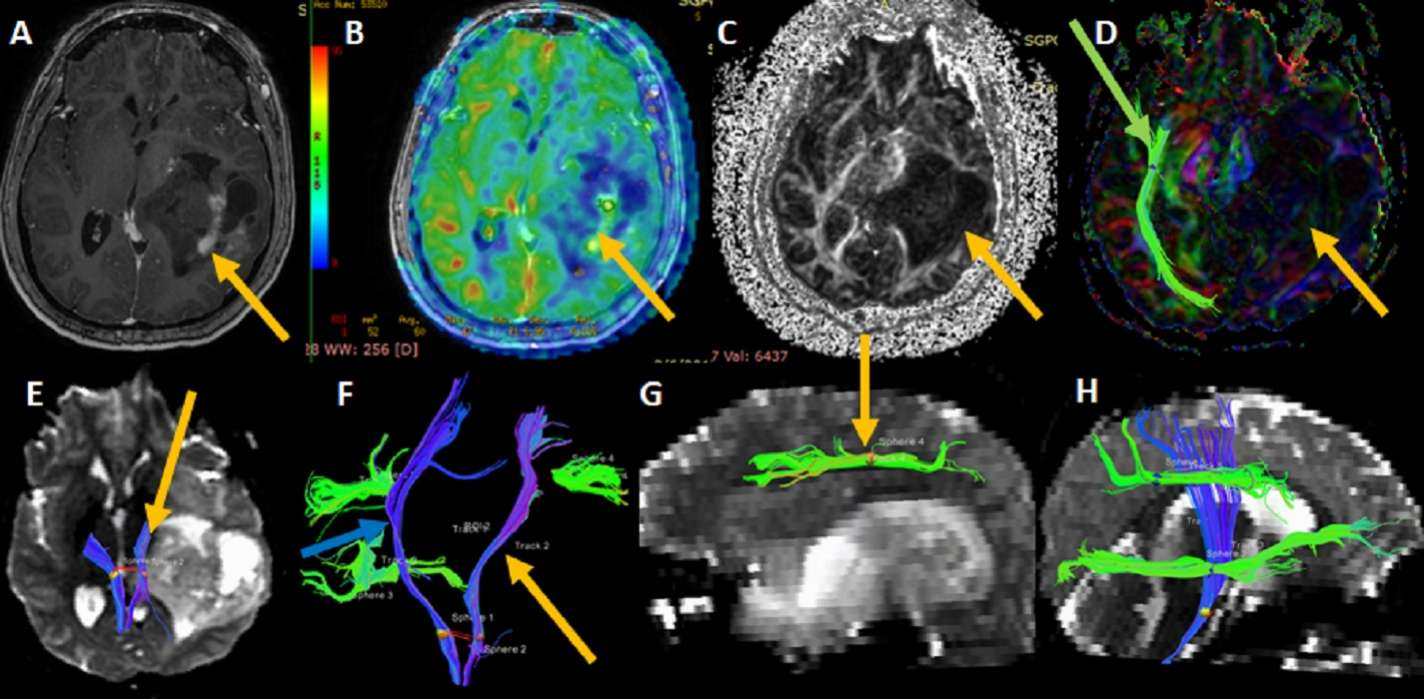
A 38-year-old male with left glioblastoma T1-weighted post-contrast image (A) shows the tumor in left temporal parietal region. Arterial spin labeling image (B) shows focal area of increased cerebral blood flow (arrow) within the enhancing area. Fractional anisotropy (FA) map (C) shows an intralesional decreased FA (arrow). Color-coded map (D) shows intralesional white matter tracts disruption of left inferior frontal-occipital fasciculus (IFO) tract (yellow arrow). The right IFO (green arrow) is intact and shown for comparison. 3-D fiber tractography (E, F) show blue-colored left CST (arrow) in close relation to lesion, and compressed and deviated anteromedially by the mass lesion. The right corticospinal tract (CST) is also displaced because of midline shift and mass effect. (G) The left green-colored arcuate fasciculus (arrow) is not affected by the lesion. (H) Normal intact left side fibers CST, IFO and AF (arcuate fasciculus) are seen.
